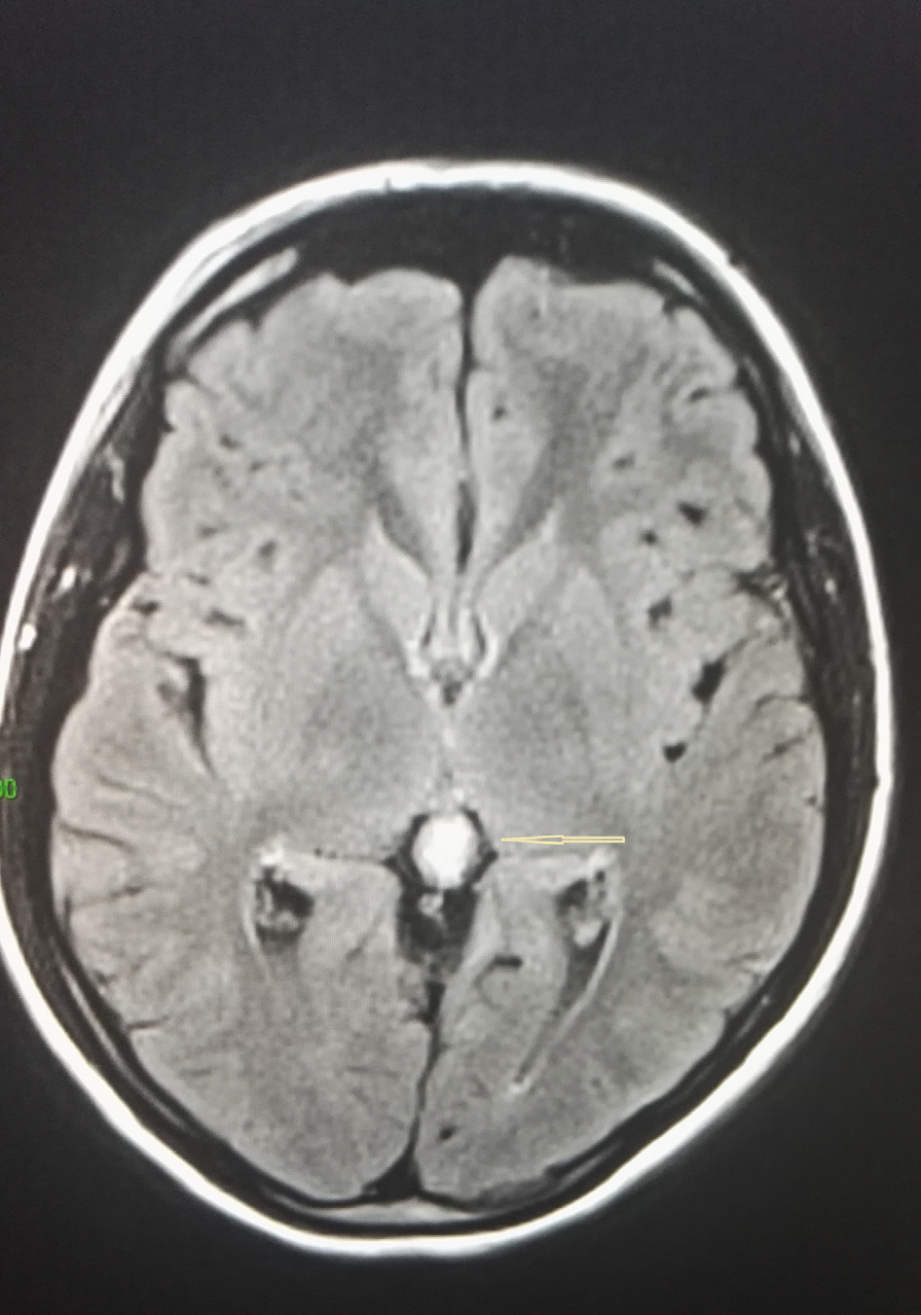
Brain MRI showing 7–8 mm pineal cyst.
